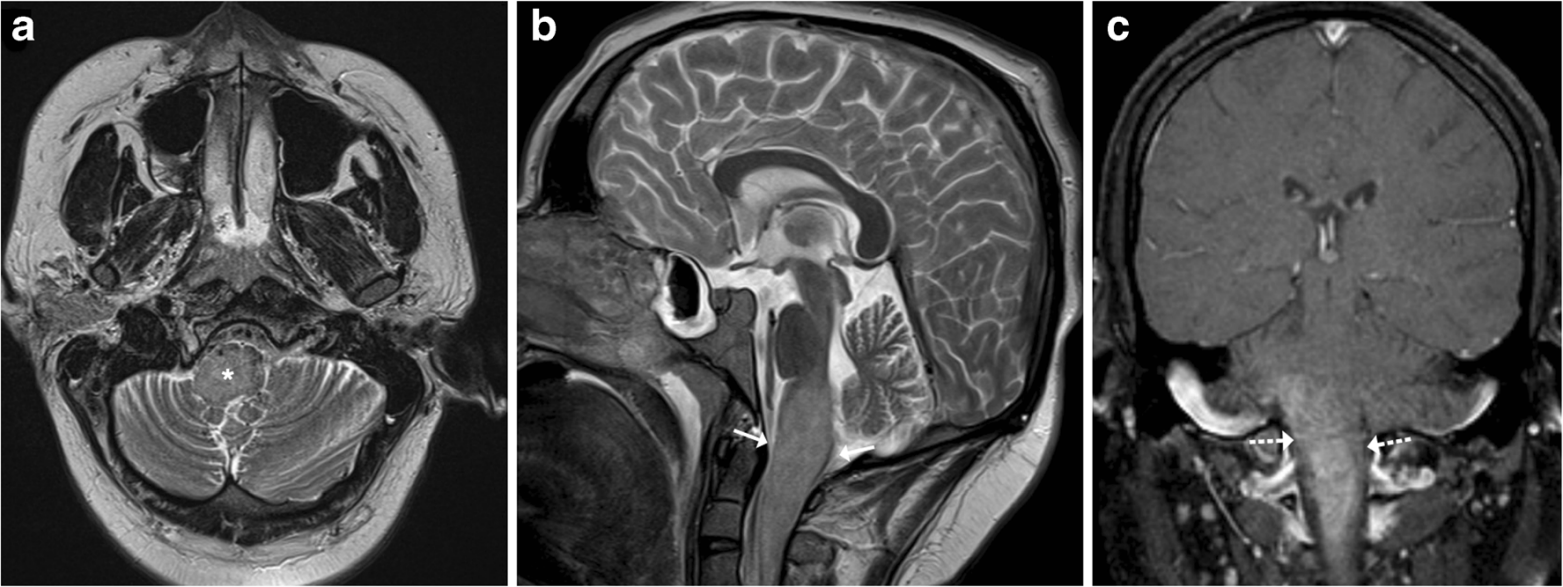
A 15-year-old male with altered headache and lower cranial nerve palsies: a–b Axial and sagittal T2-weighted image reveals an ill-defined hyperintense expansive lesion involving the medulla (*), which is extending caudally to involve the cervical cord (*arrow*). c Post contrast the lesion shows homogeneous enhancement (*dotted arrows*)
